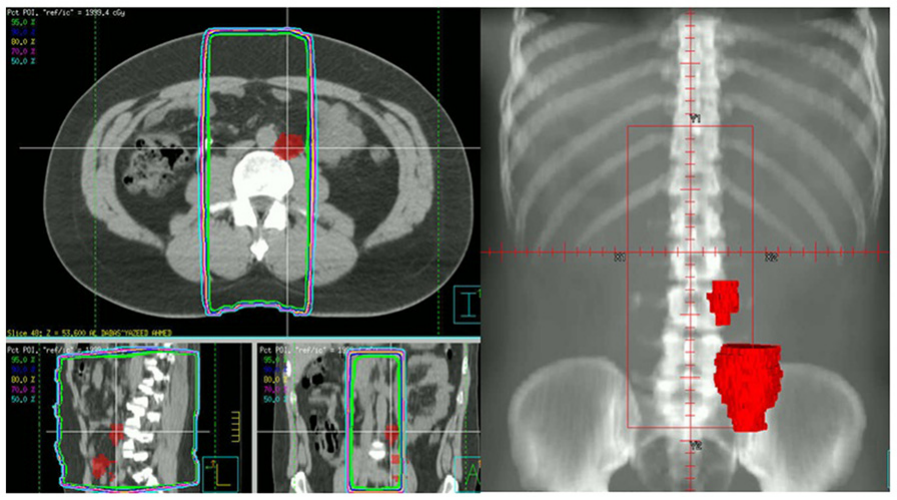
Co-registration of CT scan demonstrating recurrence with the panning CT scan; recurrence is in-field.
